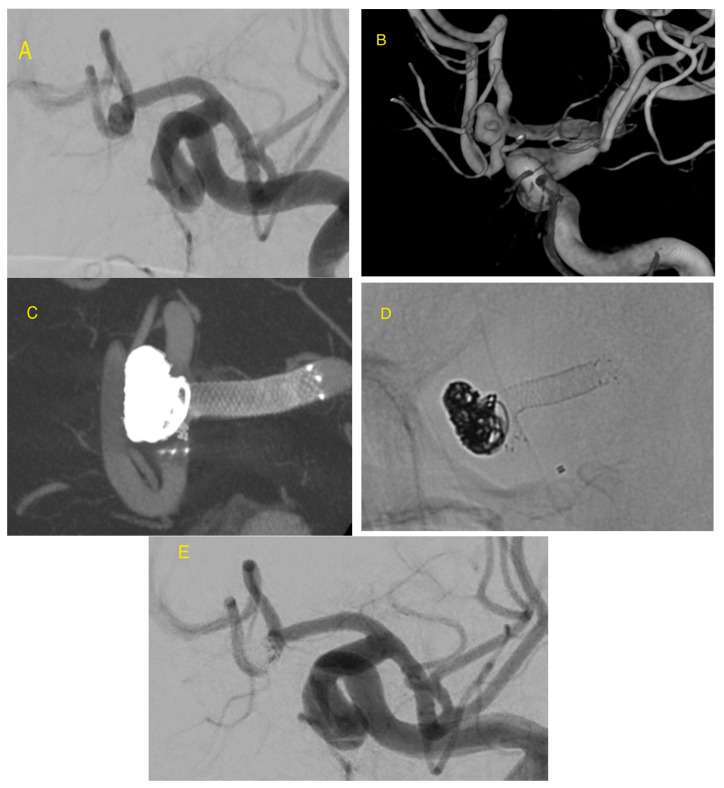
AComA wide-necked aneurysm is seen on DSA (A). Three-dimensional reconstruction of a rotational angiography (B) demonstrates the complex aneurysm morphology. LVIS EVO stent assisted coiling was used to treat this ruptured aneurysm. Flat panel detector computed tomography (CT) and Maximum Intensity Projection (MIP) reconstruction (C) and X-ray picture (D) showing very flexible stent arm, angled 90 degrees, protects completely the wide neck of the aneurysm from coil protrusion. DSA shows aneurysm occlusion (E).
